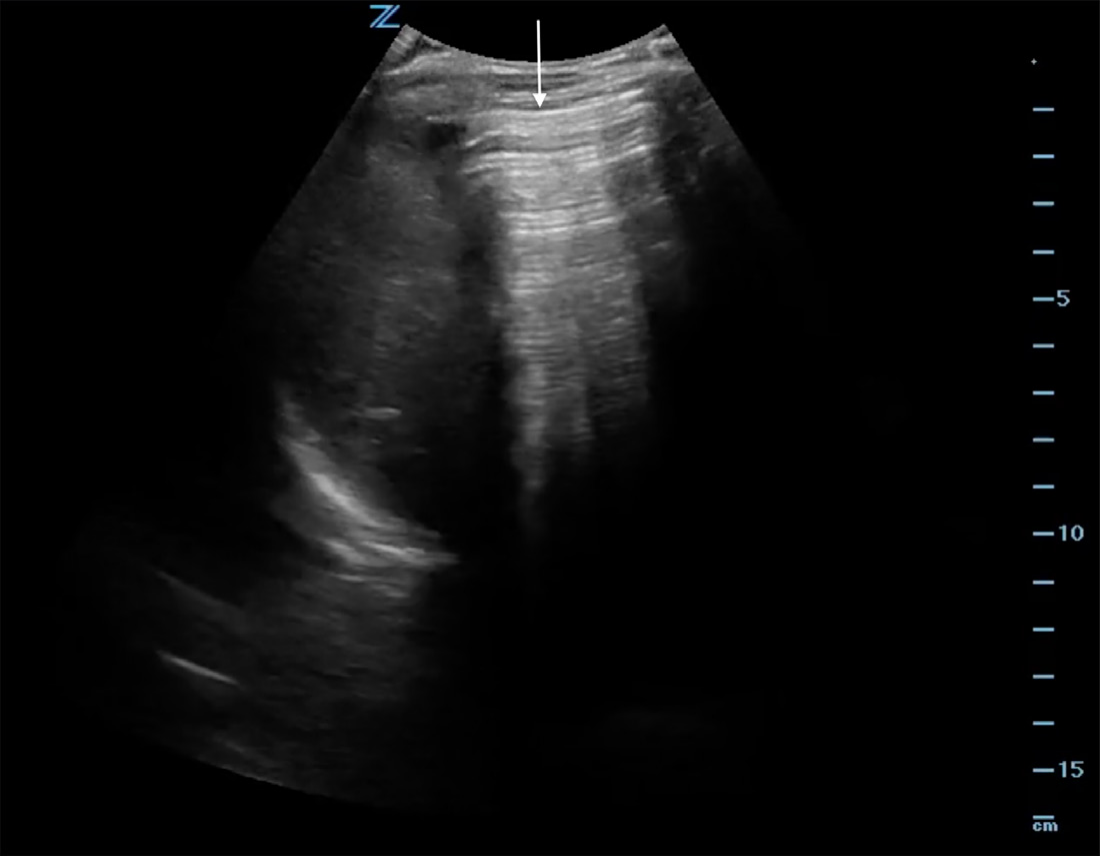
Ultrasound image from Case 2 showing enhanced peritoneal stripe sign in the right upper quadrant, indicative of free intraperitoneal air (arrow).
